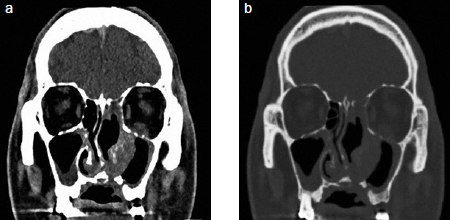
Computed tomography view showing bony erosion of the left hard palate and maxillary sinus walls. a: Soft-tissue window view; b: bone window view.
